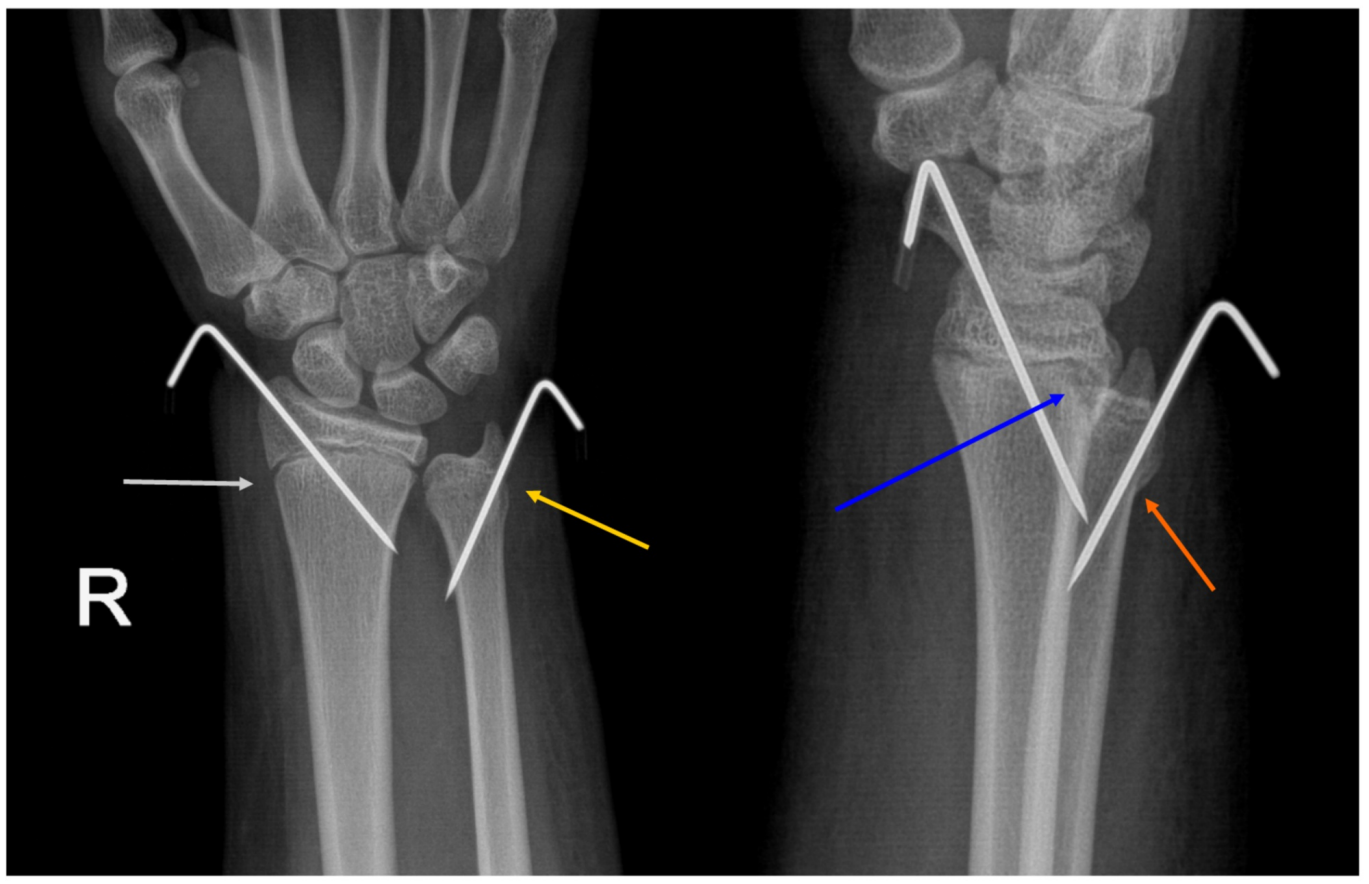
Post-operative radiographs of the right wrist showing K-wires in situ White arrow: radial physis, post reduction; yellow arrow: ulnar physis, post reduction; blue arrow: fleck sign in the radius; orange arrow: fleck sign in the ulna.
